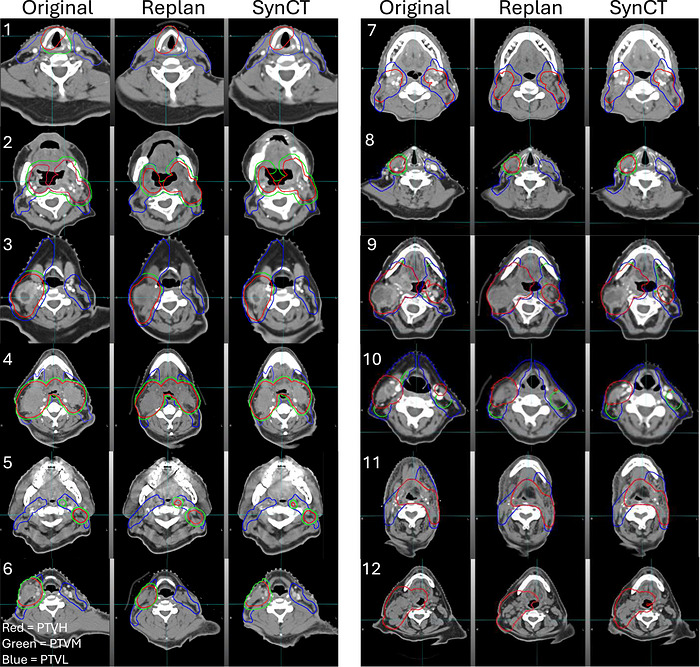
Example slice for each patient showing PTVH, PTVM, and PTVL, showing synthetic CT quality compared to replan and original CT.
